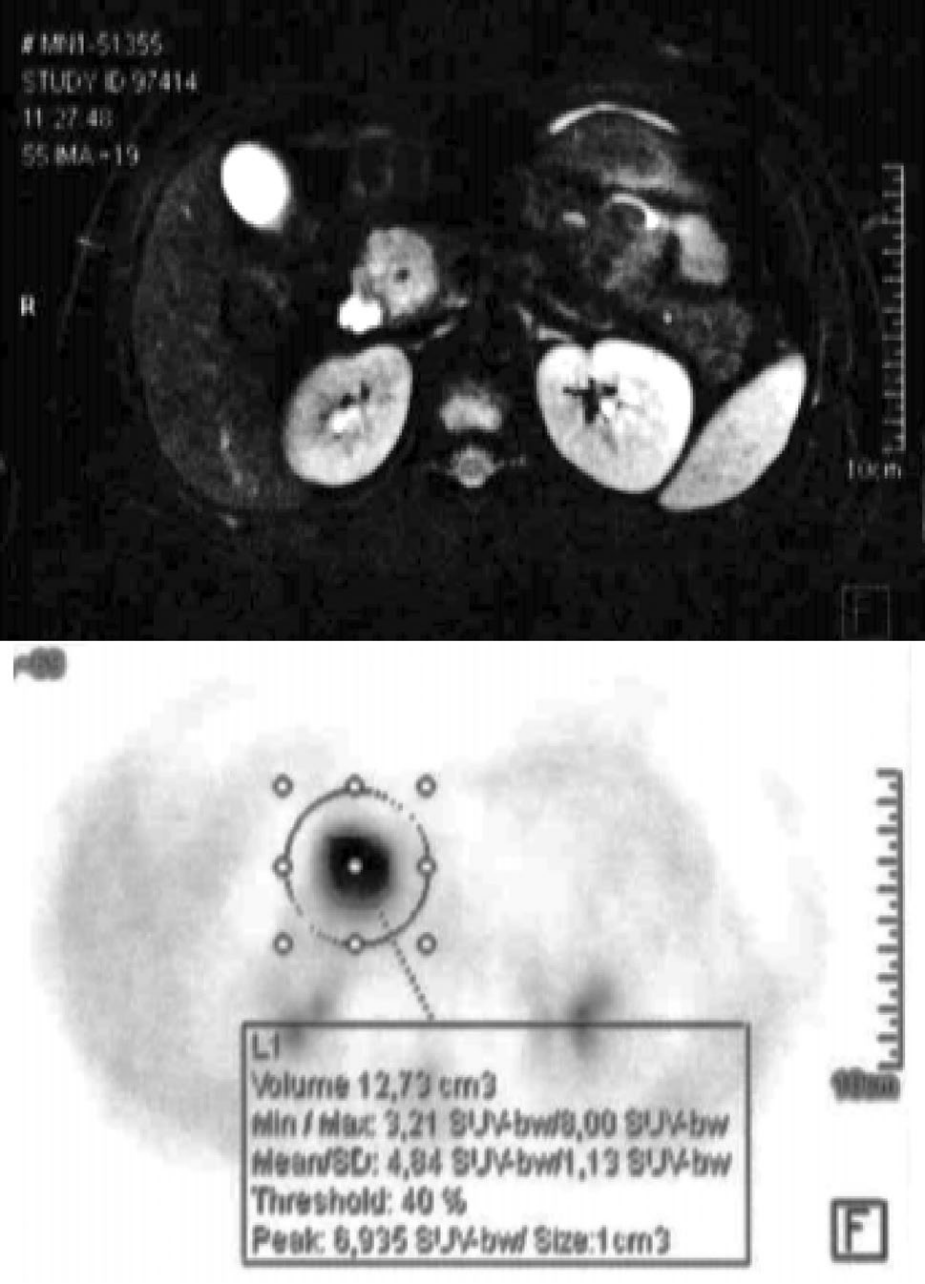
^18^F-FDG PET-MRI of case n.3: 2.9 cm in size SPN of the pancreatic head, with an intense tracer uptake (SUVmax 4.8)
